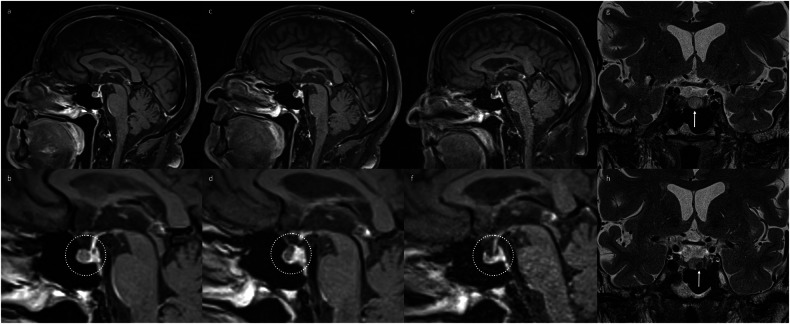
Serial sagittal post T1-weighted images of the pituitary gland (top row) and corresponding zoomed panels (bottom row, dotted circles) in a patient who had received four cycles of combination ipilimumab and nivolumab for metastatic melanoma, demonstrating an atypical appearance of hypophysitis, related to the presence of a pre-existing Rathke cleft, visible as a round non-enhancing area at the anterior aspect of the pituitary gland prior to ICI therapy (a, b). Despite the Rathke cleft cyst, enlargement of the enhancing pituitary tissue is visible (c, d) and leads to a change in the configuration of the cyst. Later (e, f), both the cyst and the pituitary tissue itself appear smaller than at baseline. The findings of hypophysitis occurred while on low-dose oral corticosteroids for a treatment-induced rash and resolved without additional treatment. Dedicated coronal T2-weighted images obtained at baseline further characterise the anterior Rathke cleft cyst, showing it to be predominantly T2-hyperintense (g), except for a T2-hypointense intracystic nodule (h), consistent with a Rathke cleft cyst [30]
